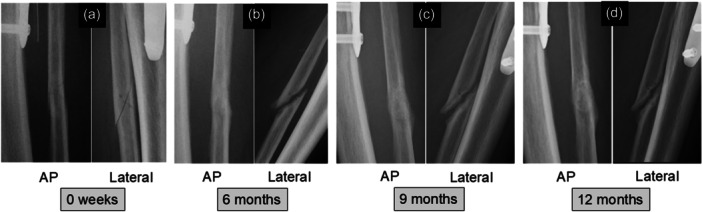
A 58‐year‐old woman with asymptomatic non‐union. The anteroposterior (AP) and lateral radiographs taken immediately after surgery show that adequate contact between the osteotomy ends was achieved during surgery. However, callus formation remained insufficient throughout the post‐operative period.
